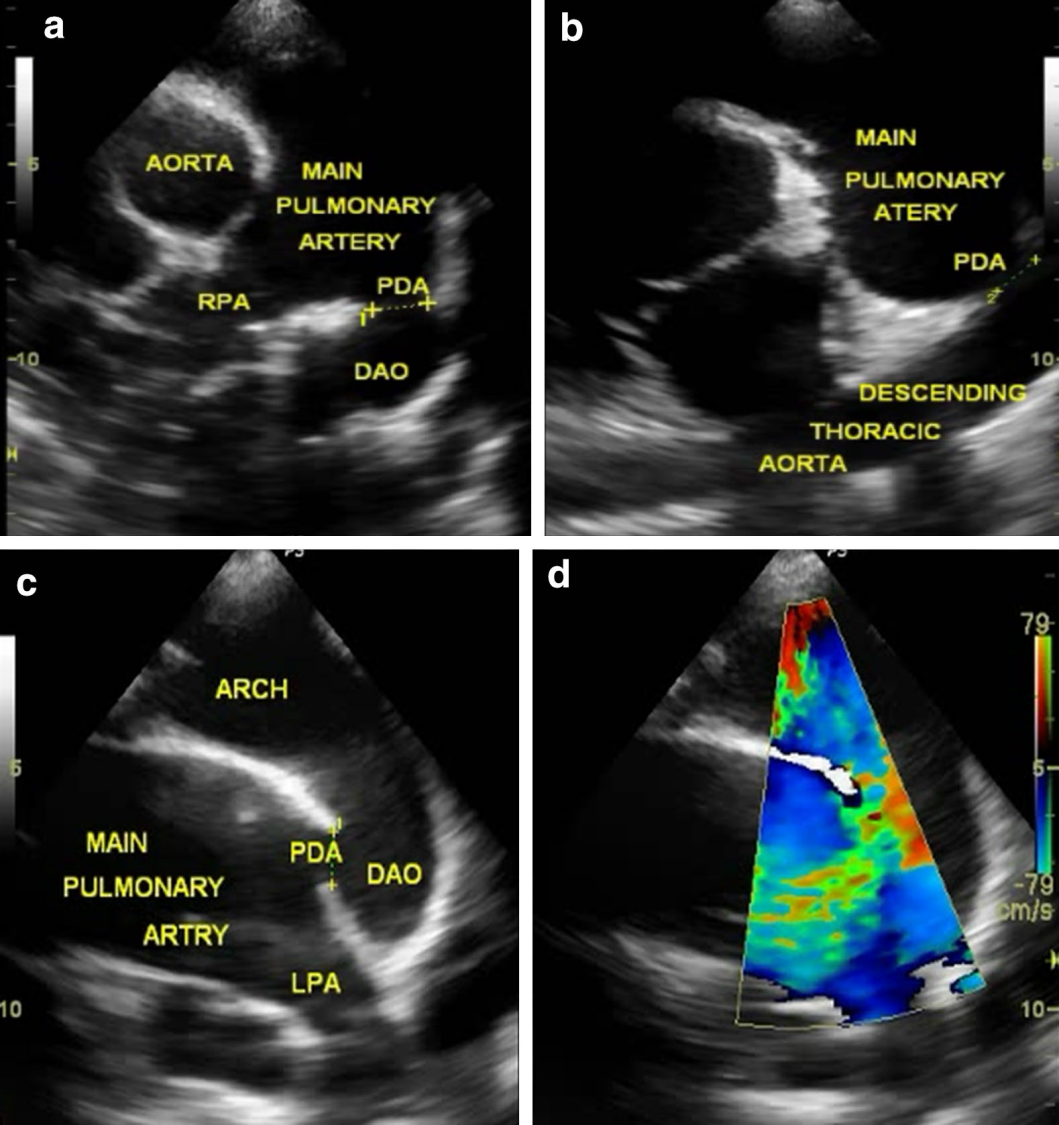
Echocardiographic frames showing large window-type PDA (a Parasternal short axis view; b Modified duct-cut view; c Suprasternal view; d Suprasternal view with *color* flow)
